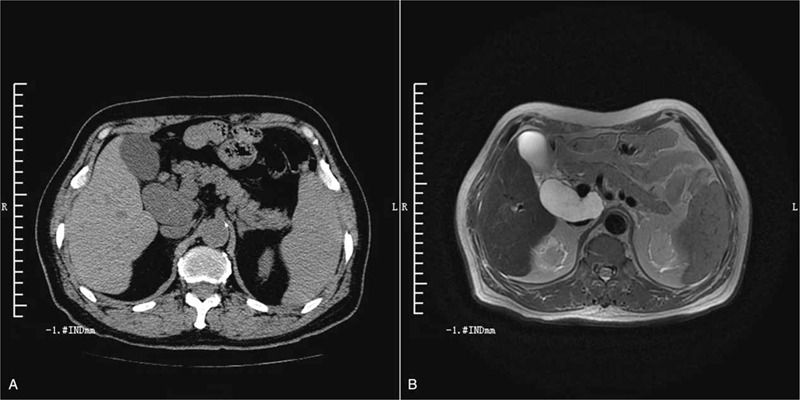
Imaging findings. A. Abdominal CT scan showed a cystic mass behind the head of pancreas. B. Abdominal MRI showed the mass surrounding the portal vein.
